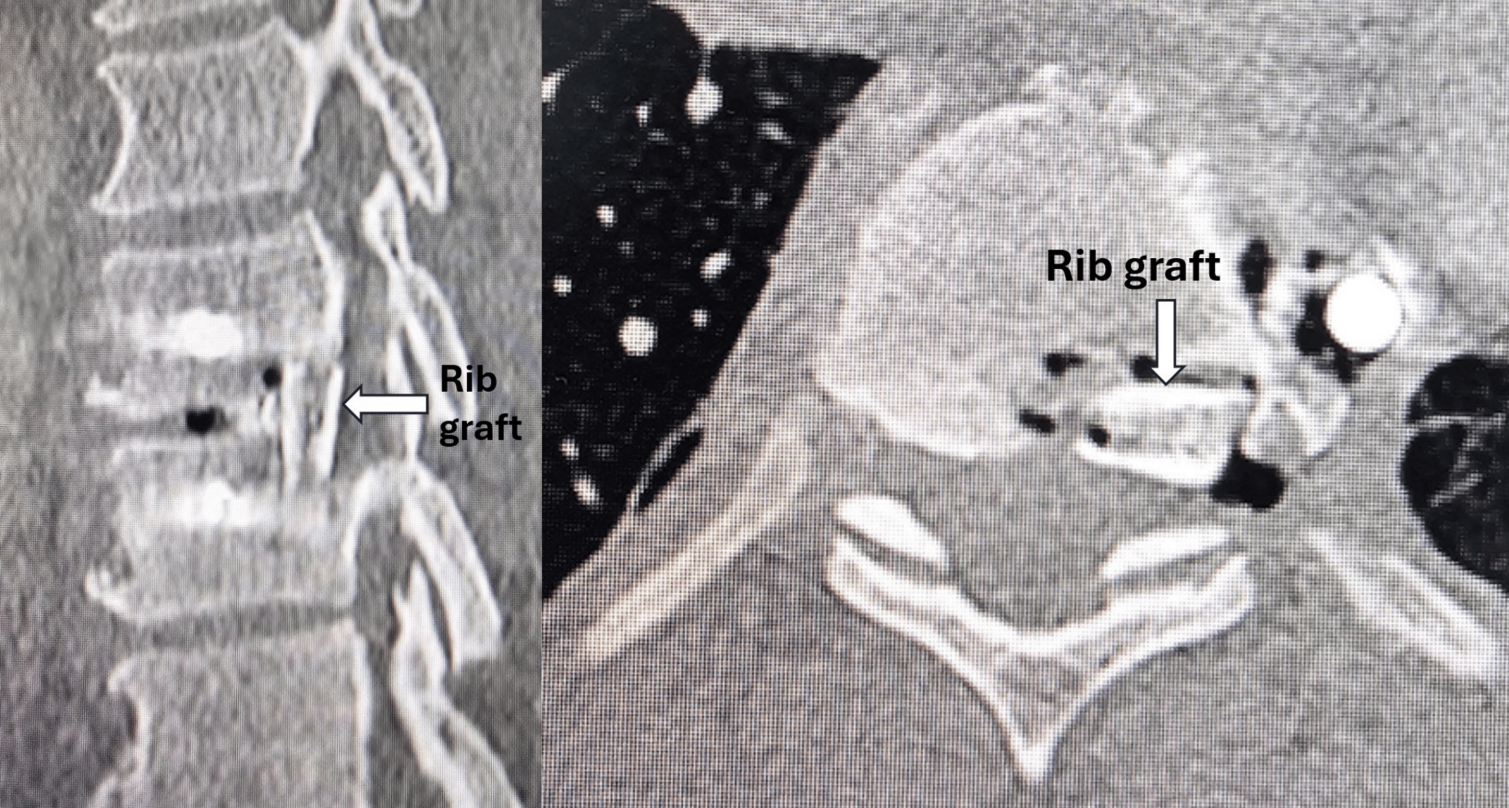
Postoperative CT scan demonstrated that the rib graft filled the void.
